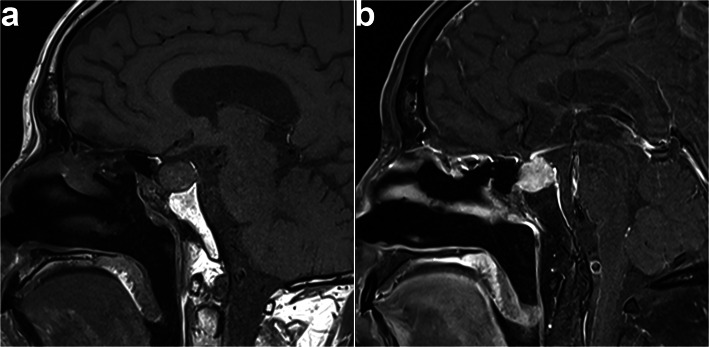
a T1-weighted MRI in the sagittal plane showed a space-occupying mass in the sellar region. b T1 postcontrast MRI in the sagittal plane showed heterogeneous enhancement in the mass
